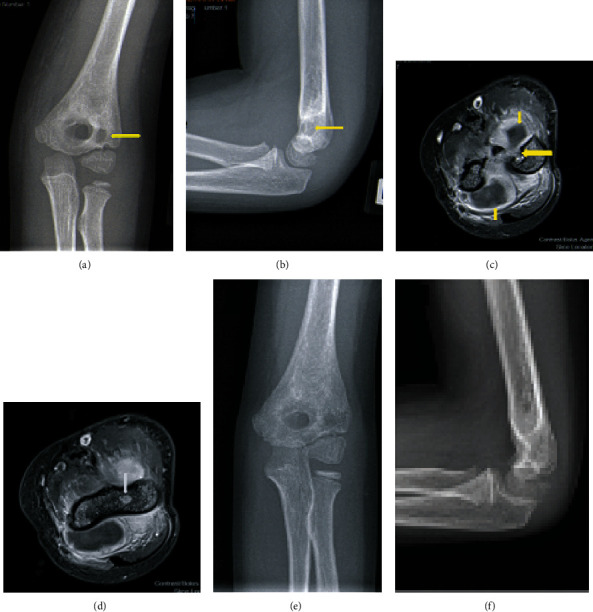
(a, b) AP and lateral x-rays showing a distal humeral lucency concerning for chronic osteomyelitis. (c, d) Axial T1 post contrast MRI. (c) The large arrow denotes nidus of infection. (d) The large arrow denotes anterior cortical disruption. The small arrows denote purulent fluid collection in elbow joint. (e) and (f) are radiographs one year after irrigation and debridement.
